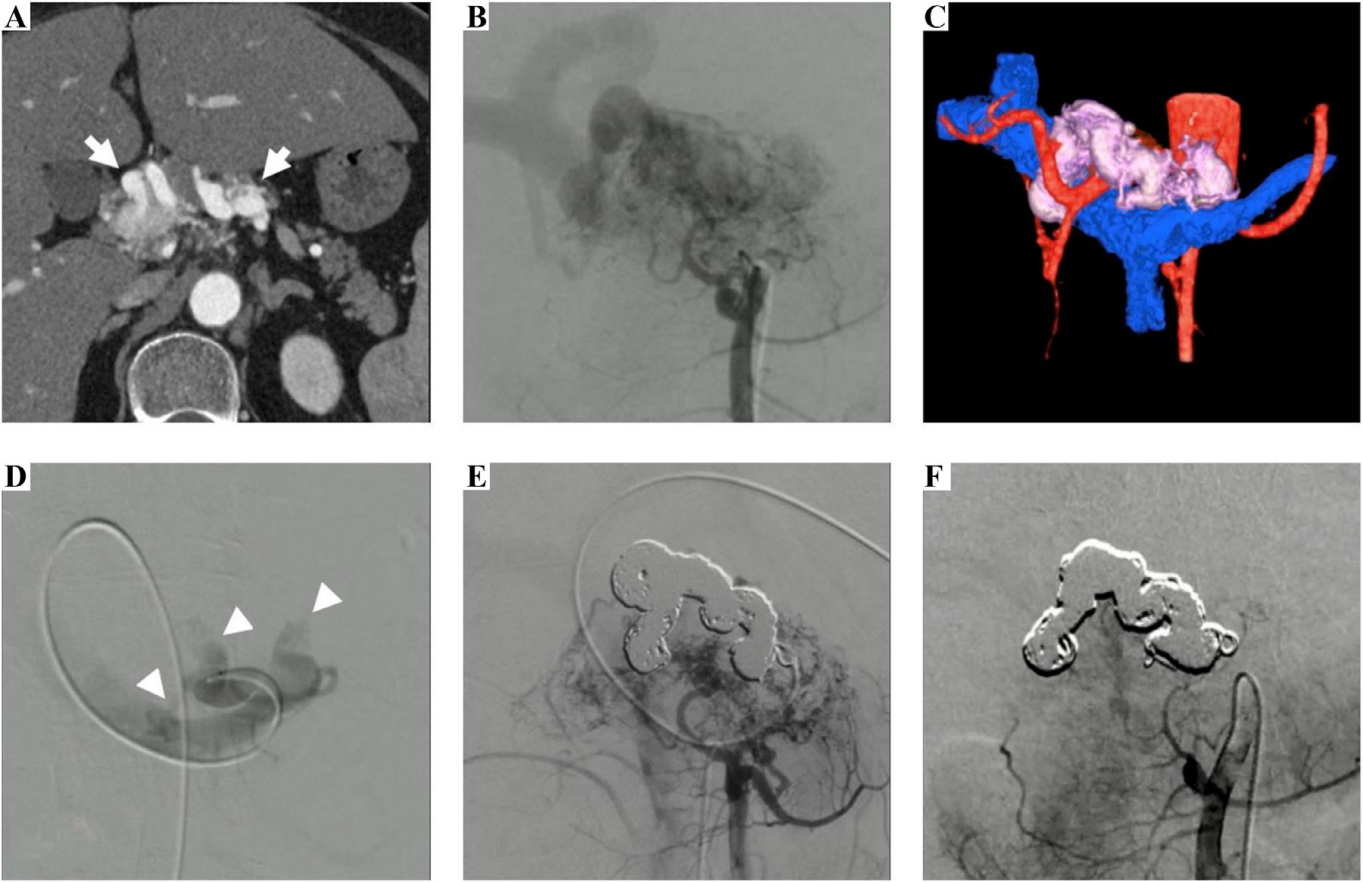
A 54-year-old female. (A) CT tomography revealing abnormal pancreatic vessels (white arrows). (B) Superior mesenteric arteriography revealing a type IIIB pancreatic AVM with multiple feeding arteries draining into the aneurysmal vein trunk that drains into the portal vein. (C) 3D rendering of the CT images. (D) Portal venous access and selective angiography of the aneurysmal vein trunk revealing the three main draining veins (white arrowhead). (E) Regional packing coil embolization of the entire aneurysmal vein trunk. Superior mesenteric arteriography after embolization revealing no residual aneurysmal vein trunk, a portion of residual P-AVM, and a decrease in the early portal venous appearance. (F) Superior mesenteric arteriography revealing further decrease in the feeding arteries and nidus after four months.
